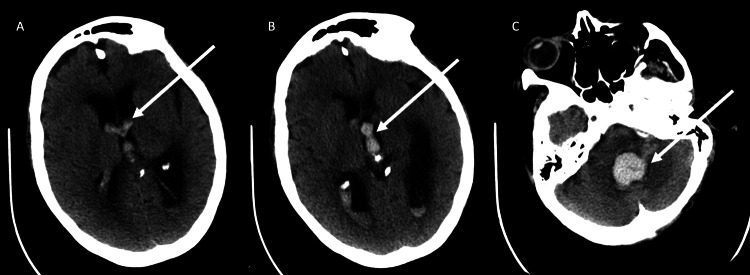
Axial cuts, non-contrast CT of the head showing intraventricular hemorrhage. (A) IVH in the fourth ventricle. (B and C) IVH and hydrocephalus in the third and bilateral lateral ventricles. White arrows point out IVH.
